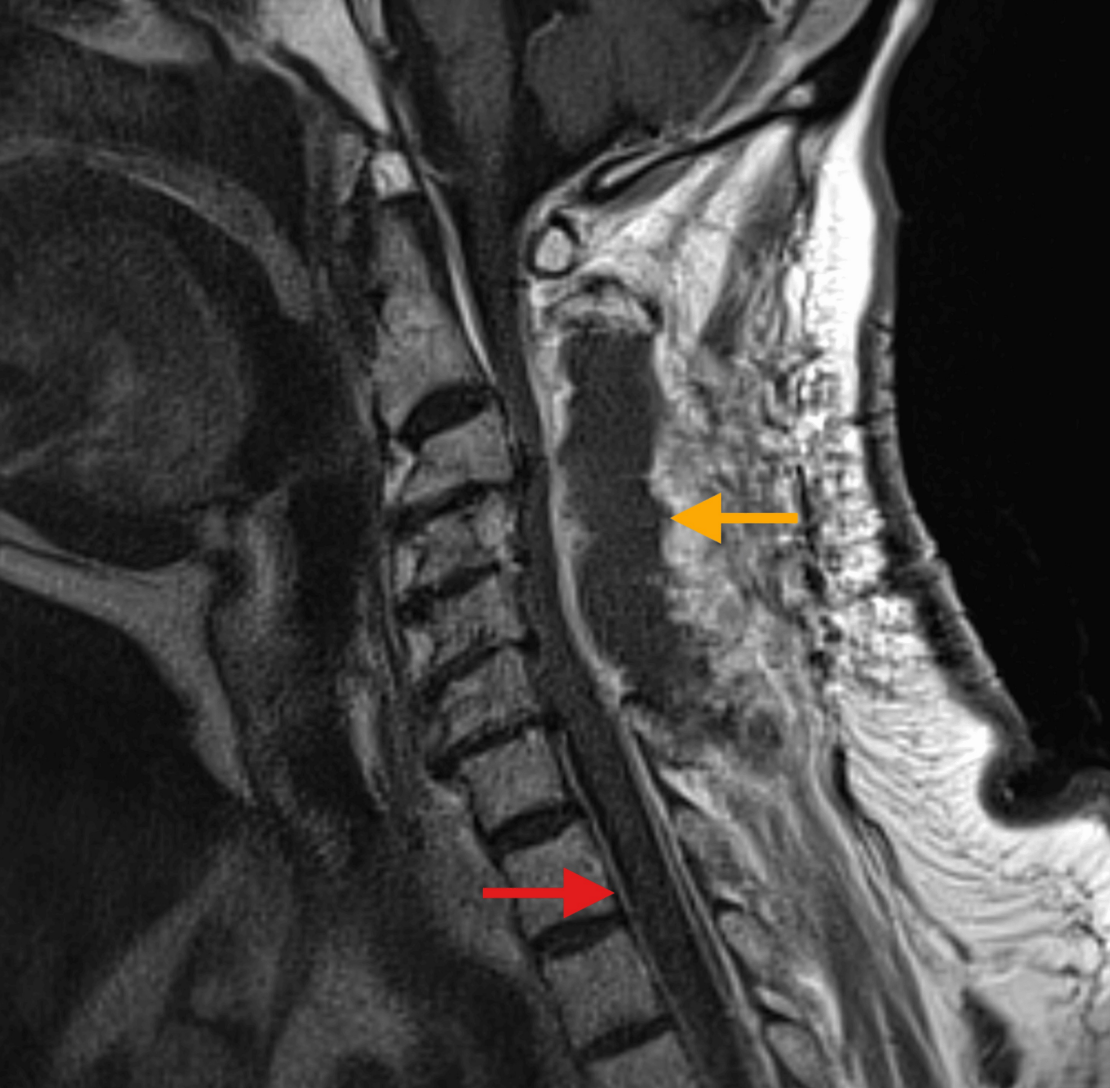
MRI cervical spine with contrast, T1-weighted Dural enhancement of the cervical spinal canal (red arrow) and dorsal epidural non-enhancing fluid collection (orange arrow).
